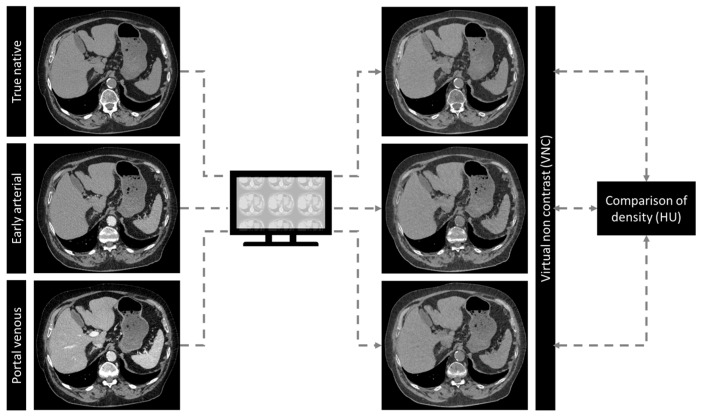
Principles of virtual non-contrast (VNC) reconstructions. Patients with triphasic photon-counting CT (PCCT) scans of the abdomen (true non-contrast, early arterial and portal venous) have been enrolled. VNC reconstructions have been performed from true non-contrast, early arterial and portal venous contrast phase and compared to true non-contrast.
